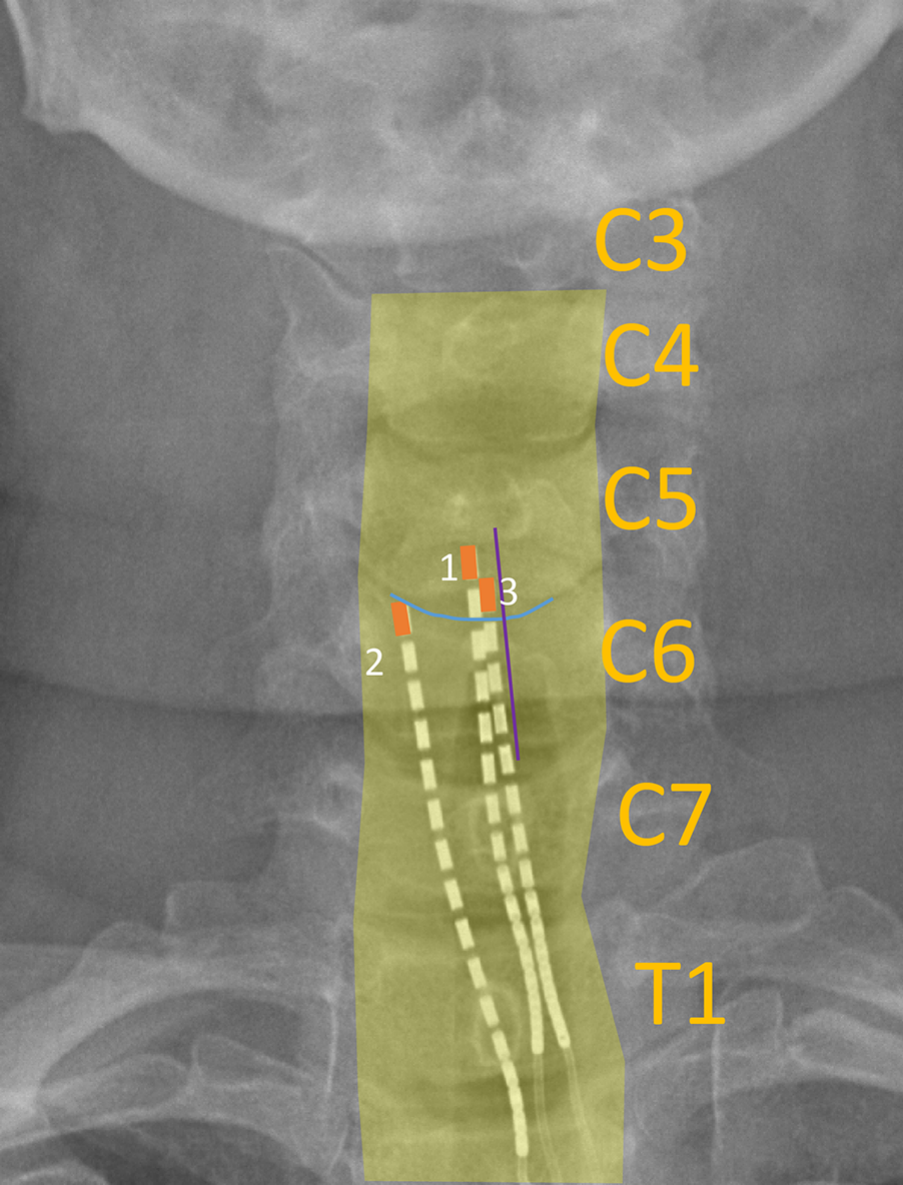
Example x-ray image labelled with landmarks used to calculate lead migration across weeks. Yellow bordering: outline of vertebral bodies; blue line: end plate (superior end plate of C6); purple line: bisection of spinous process (C6); orange box: outline of most rostral SCS contact on each lead.
